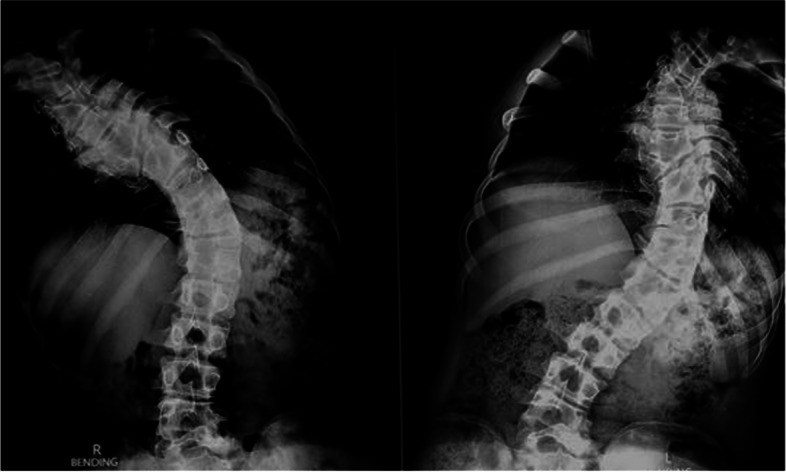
Pre-operative standing, full length lateral bending xray for scoliotic curve assessment showing a stiff curve not showing significant correction by bending, implying a stiff congenital curve
